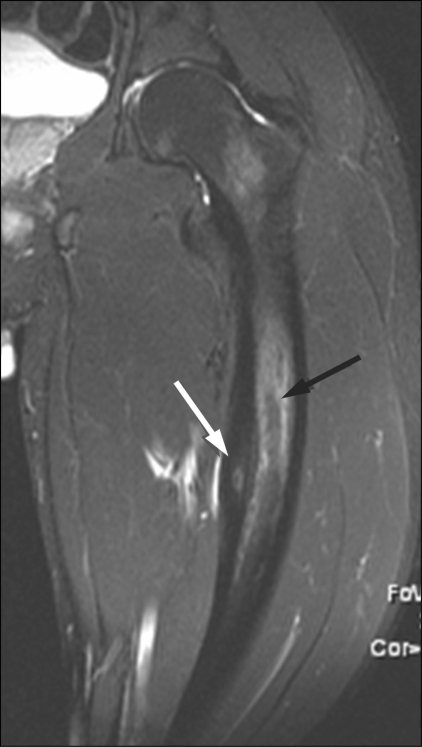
Osteoid osteoma. STIR coronal MRI shows the nidus (white arrow) along with marrow edema (black arrow)
